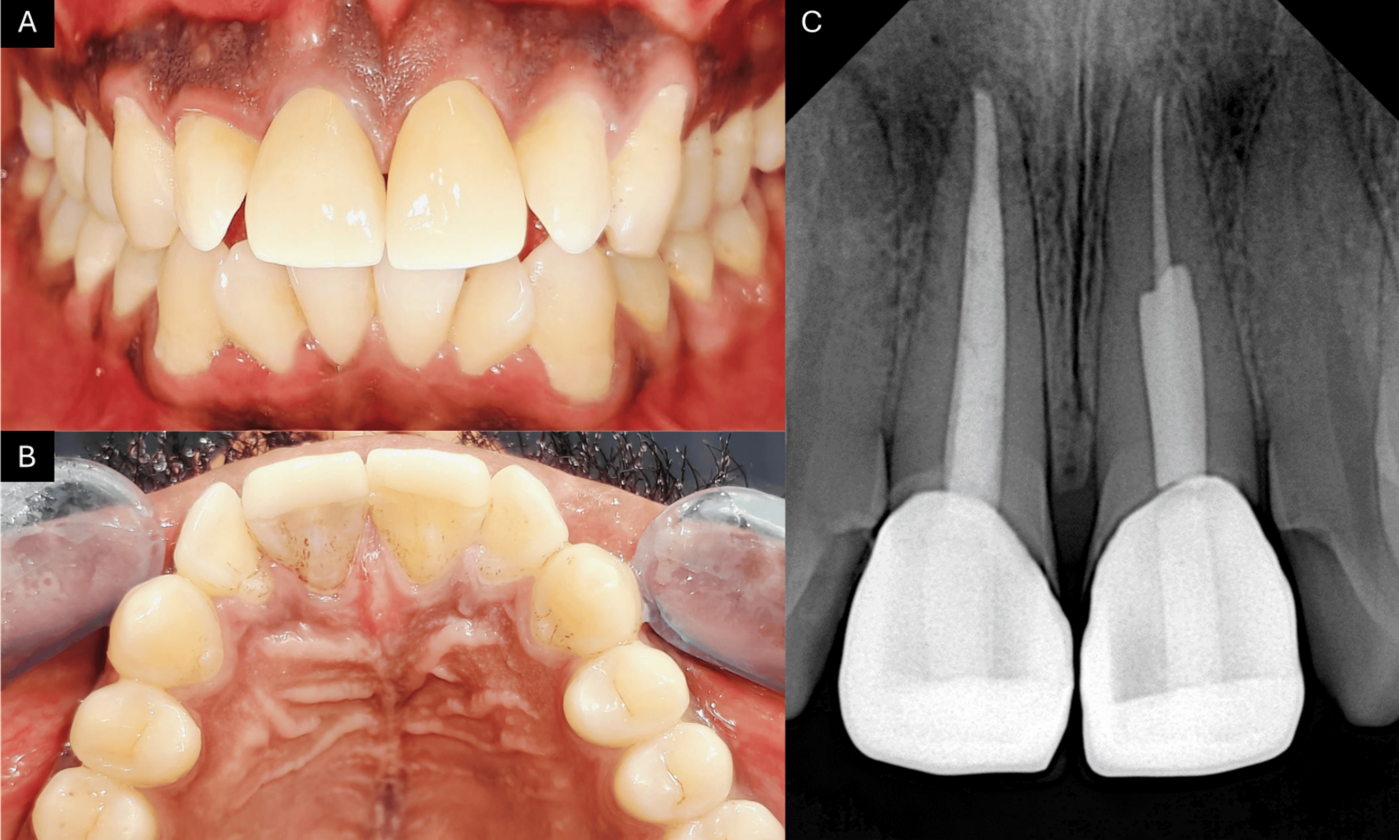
(A) Postoperative facial view, (B) postoperative incisal view, and (C) six-month follow-up radiograph
